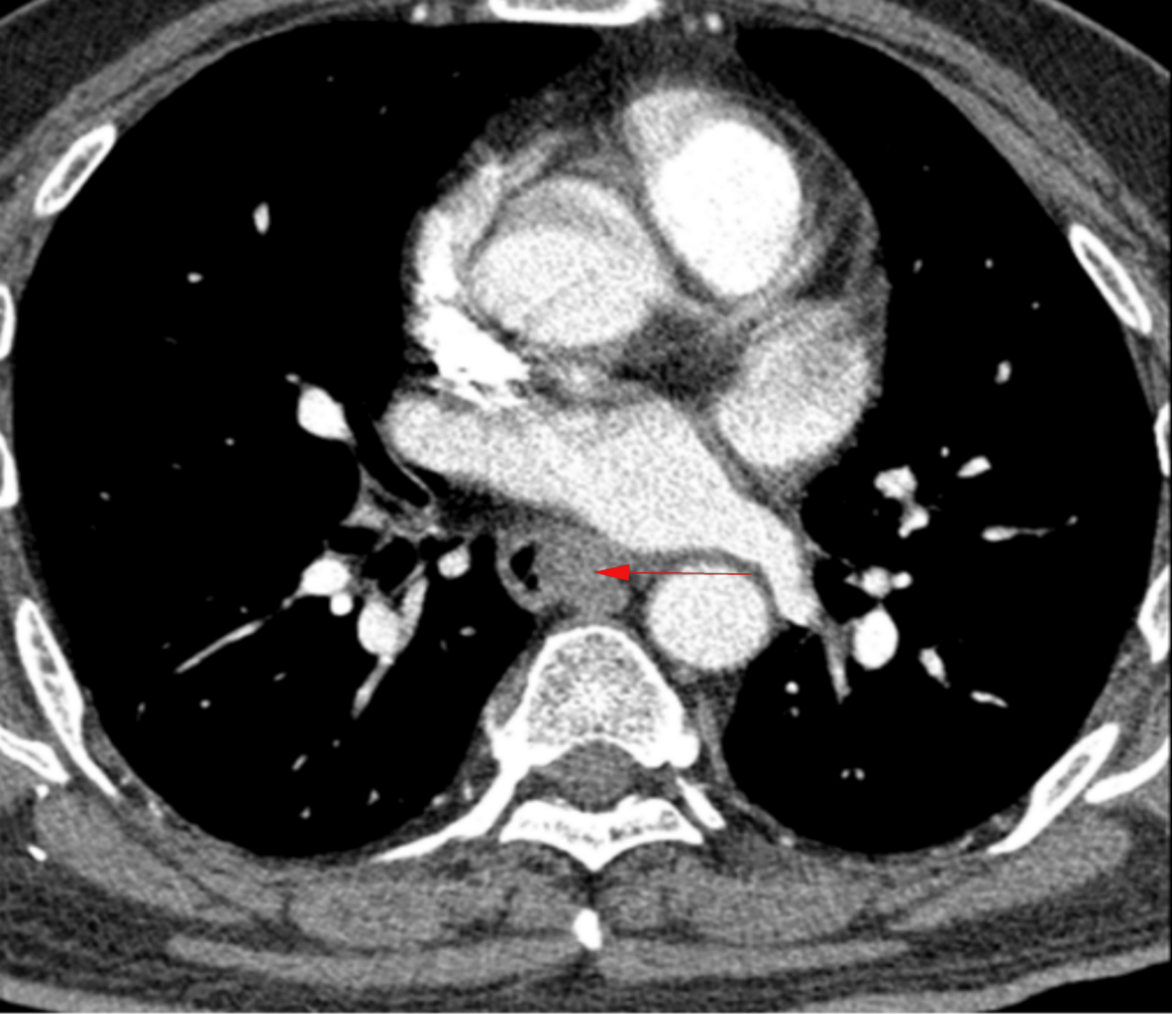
A strip of slightly high-density shadow on the left side of the mid-esophageal lumen in enhanced thoracic esophageal CT (red arrow).
